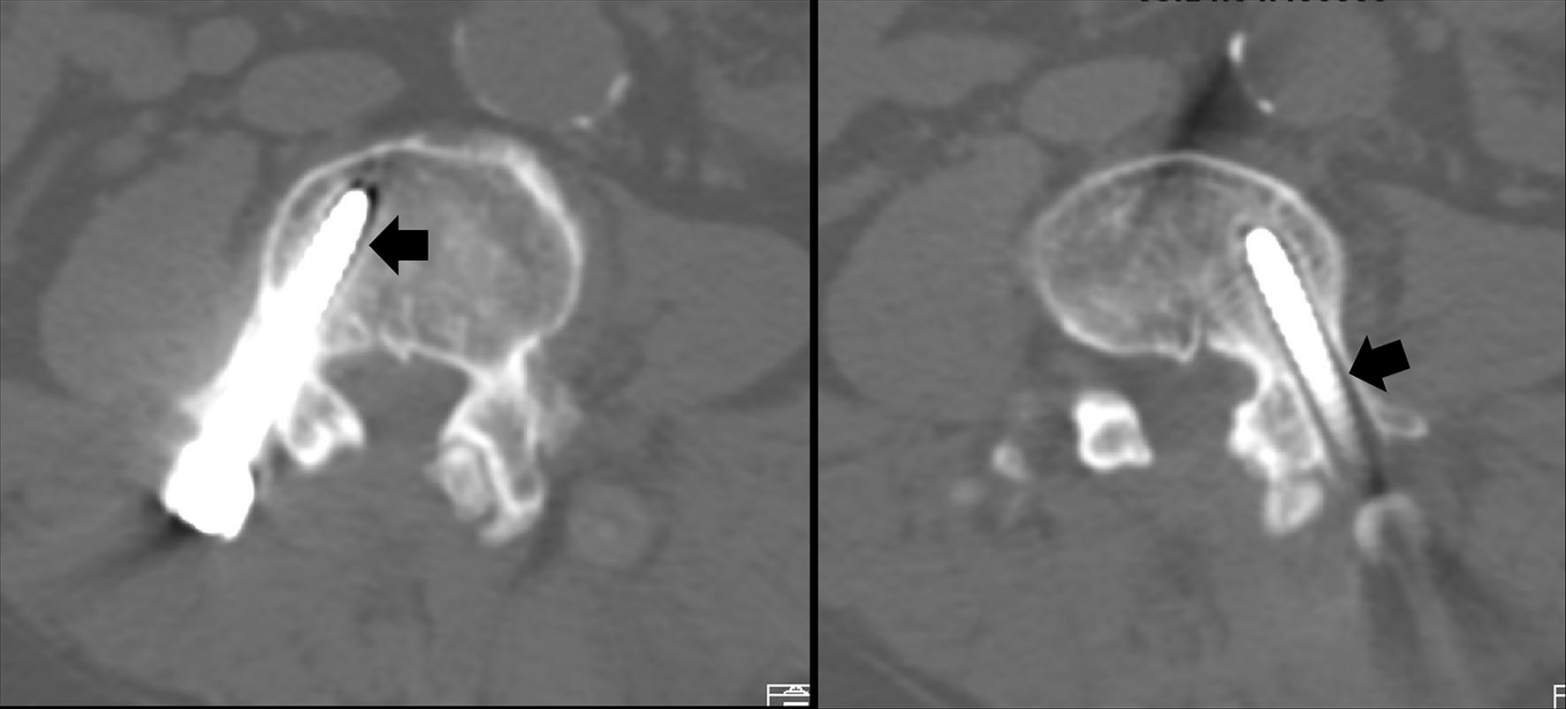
Post-operative 24 months CT demonstrated bilateral screw loosening at L4 in a 64 year-old male who underwent dynamic stabilization at L3–4 (black arrow: double halo sign), although all HU values of L4 pedicles were much higher than the mean HU values in our cohort. (L4 right pedicle HU including/excluding cortex bone: 350/276; left pedicle HU including/excluding cortex bone: 355/272; VB HU: 117).
